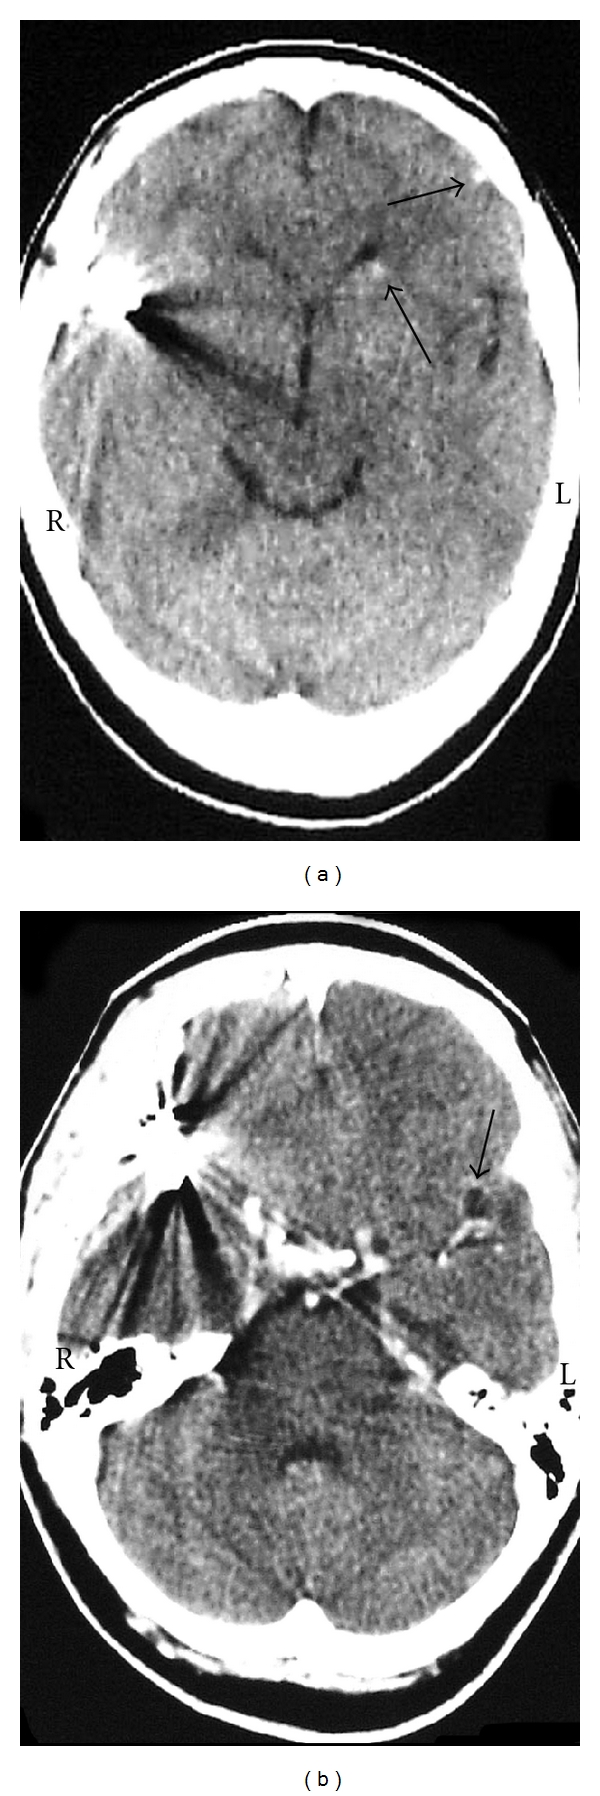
(a) CT scan with no contrast after surgery showing calcifications (arrows). (b) Cyst (arrow) enhanced after contrast. (R: right, L: left).
